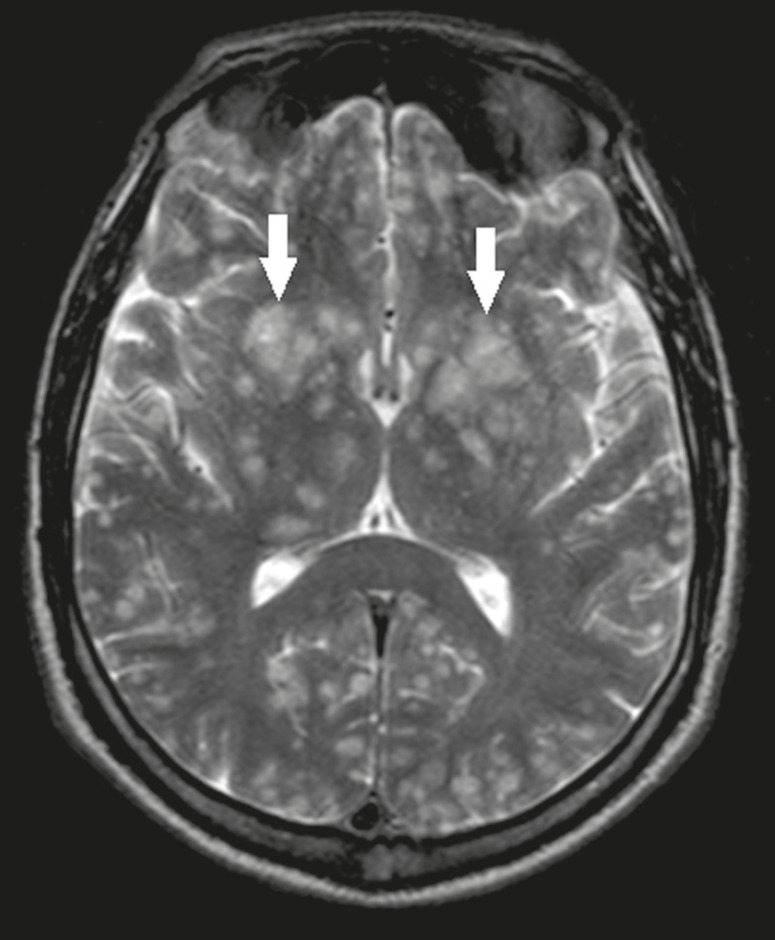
Cerebral cryptococcosis. Axial T2-weighted MRI showing hyperintense, cyst-like formations with a soap-bubble appearance, distributed throughout the basal ganglia, in the periventricular and subcortical white matter (arrows), featuring gelatinous pseudocysts.
